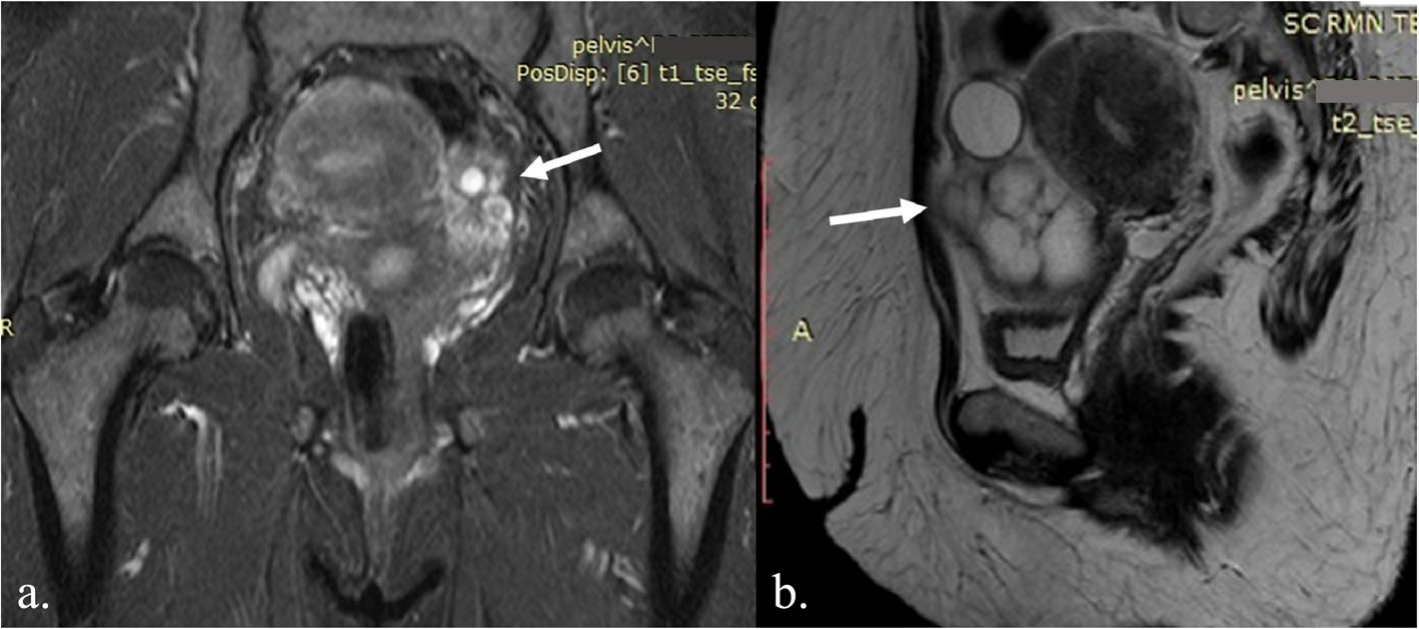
Pelvic MRI showing right adnexal mass with median development, mixed predominantly cystic structure, multiloculated, multiseptated, with reduced solid component, with dimensions of 79 × 66x72 mm (white arrow); a. Coronal MRI: T1-weighted image showing variable signal intensity for the cystic component (high and intermediate), and intermediate signal intensity for the solid component of SO; b. Sagittal MRI: T2-weighted image showing high signal intensity for the cystic component and low signal intensity for the solid component (septations and walls)
